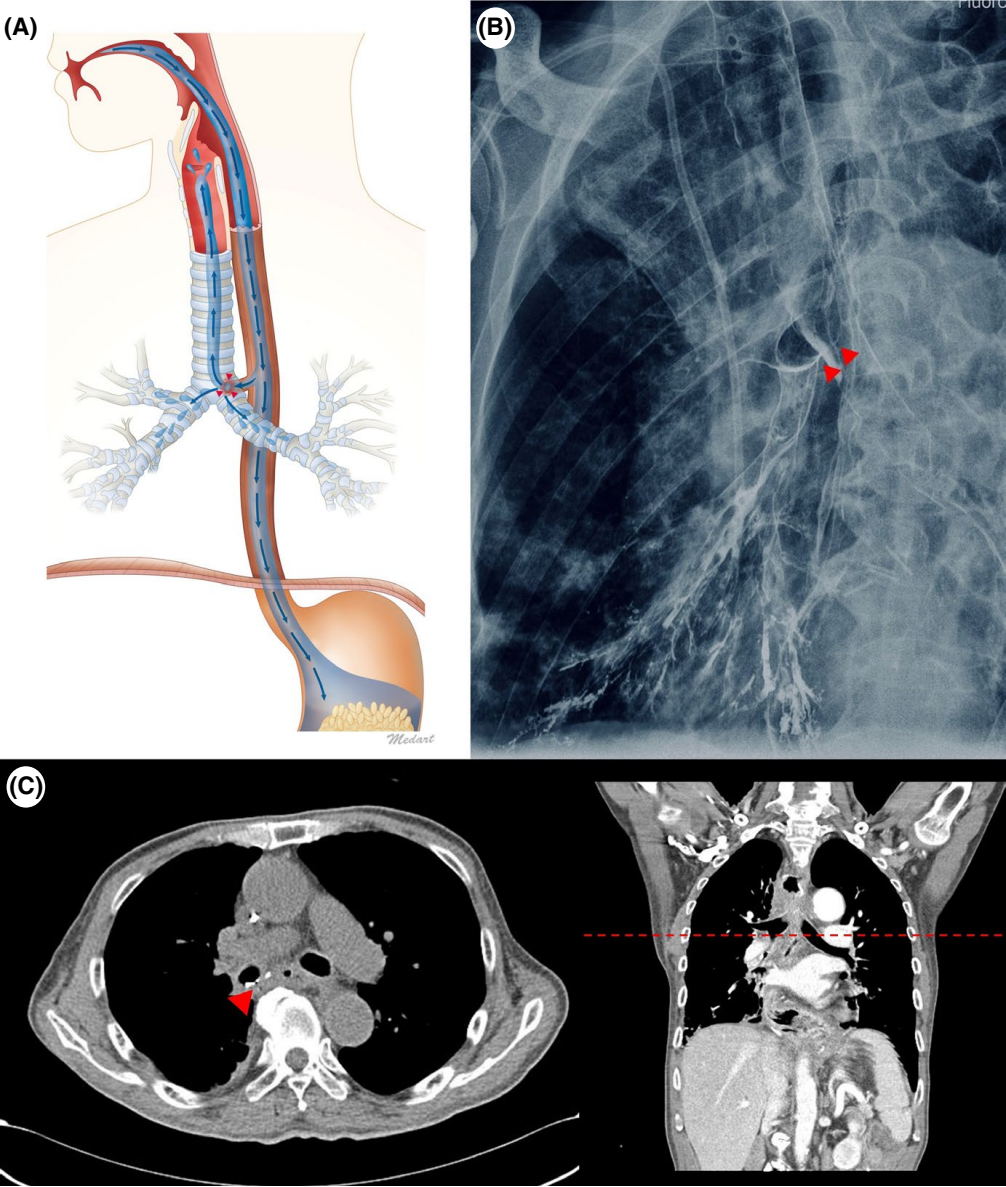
A) Figure showing that the swallowed barium into the airway system pass through small fistula (arrowhead; A), and peribronchial barium infiltration and upward reflux of barium from the trachea to the pharyngeal level (blue arrow; B) Chest X‐ray showing peribronchial barium infiltration and presence of a small fistula (arrowhead; B). C) A computed tomography confirming the presence of a small fistula (arrowhead; C)
